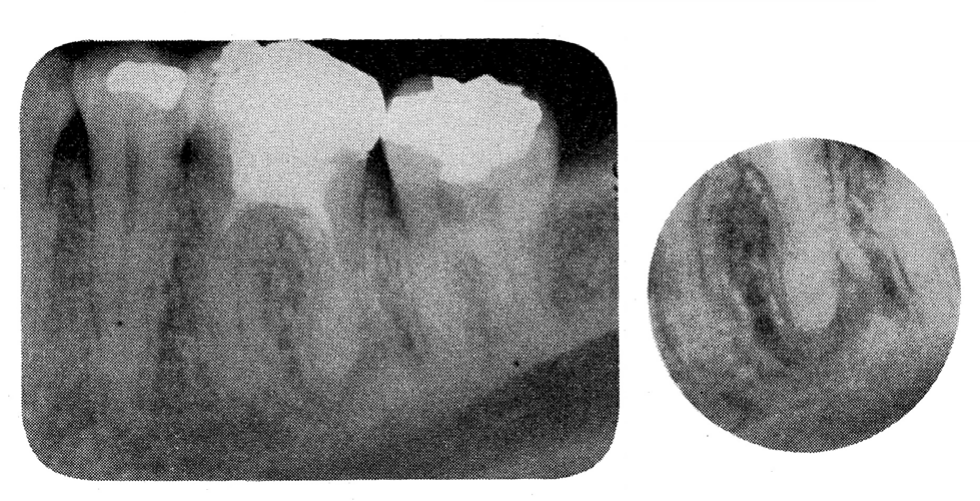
Effects of narrow collimation in intraoral radiography. *Note*. Reprinted from “Effect of narrow collimation on the image representability of periapical bone defects”, by Nishiyama S., *Dental Radiology* 1977; 17:27, Figure 10.
